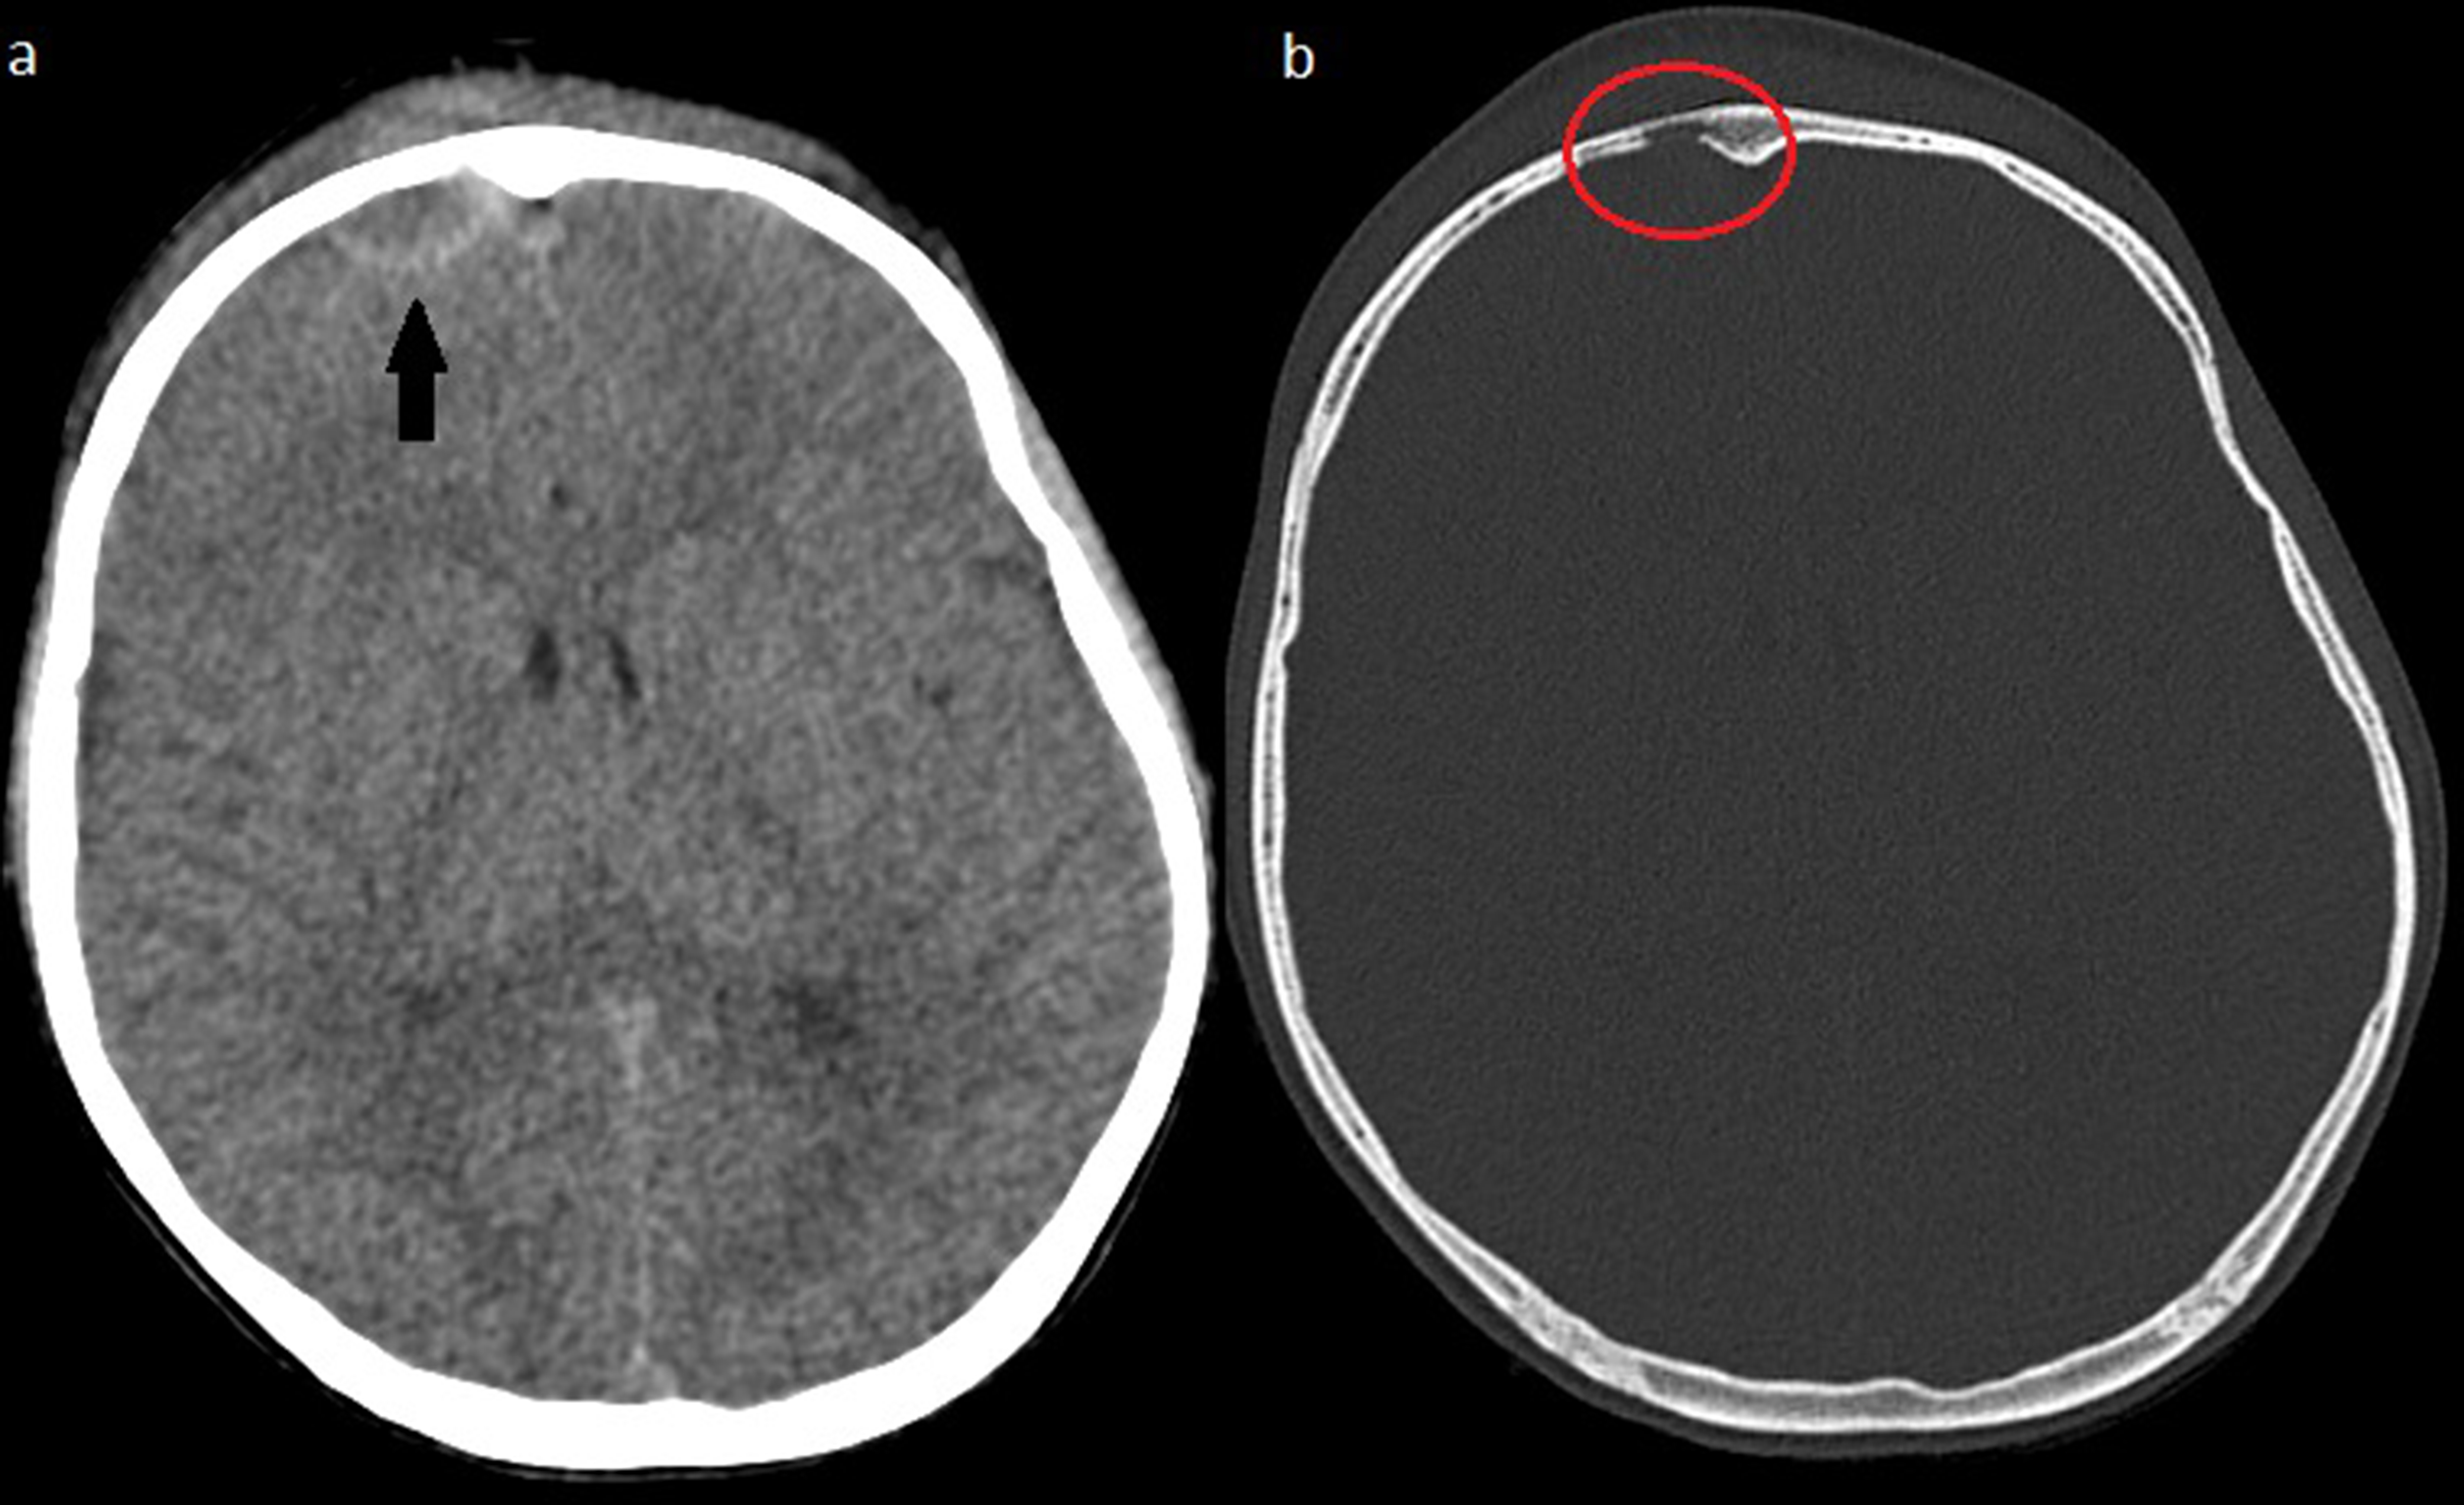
Spiral computed tomography of the head (performed after the MRI scan resulting in remainders of gadolinium contrast). a: An epidural 20 × 9 mm collection with capsular contrast-enhancement suggestive of an abscess and soft tissue swelling frontally (black arrow). b: Bone erosion internally of the frontal bone suggestive of osteomyelitis (red circle)
